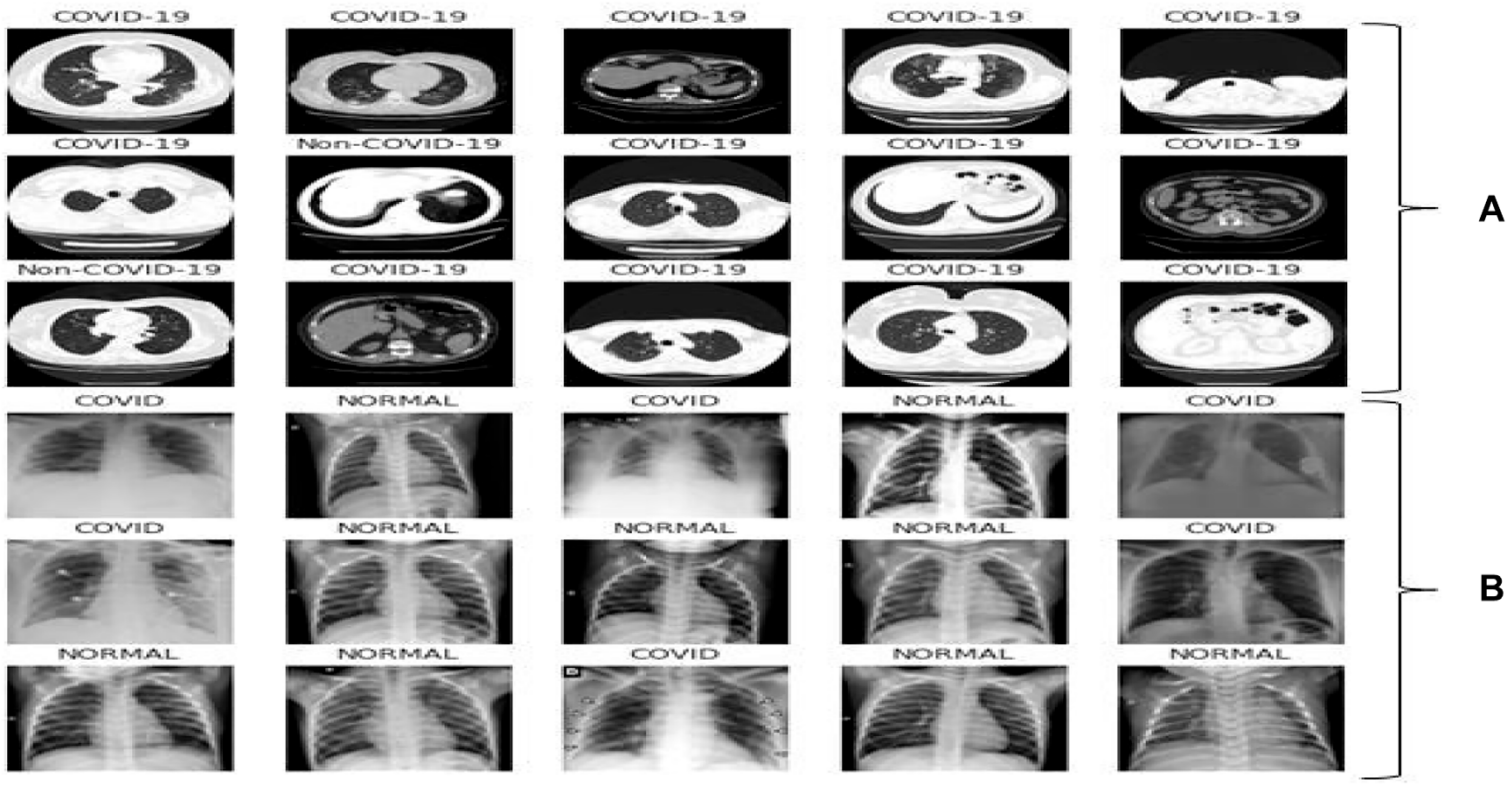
Representative samples: (A) CT scan samples from the dataset ARIA (2021), (B) X-ray samples from the dataset Siddhartha and Santra (2020).
